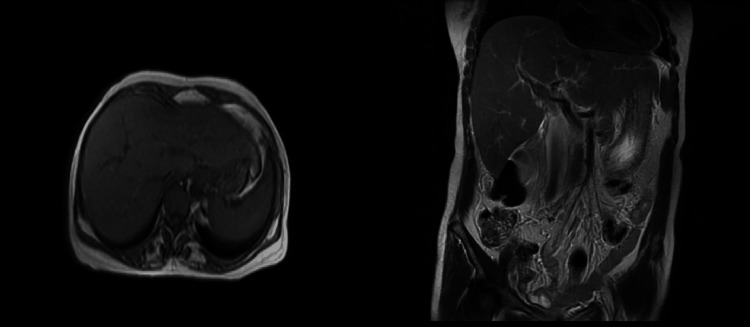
Abdominal MRI images in the T2-weighted coronal and sagittal sections indicating hepatomegaly with fatty liver
